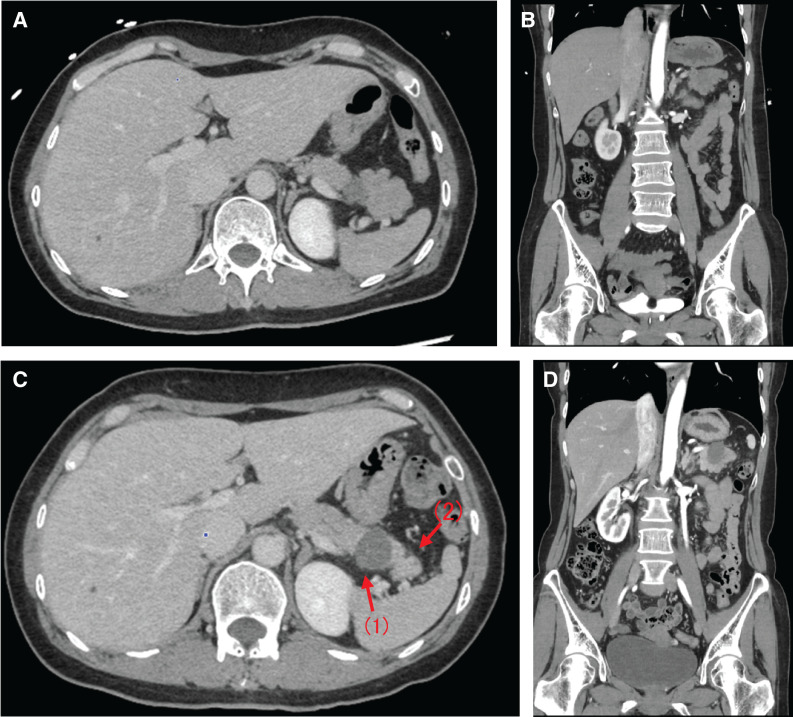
(A, B) Contrast-enhanced CT images of the pancreatic lesion at initial admission. (C, D) CT images of the pancreatic lesion on 2nd admission, revealing progression in size and morphology (arrows).
